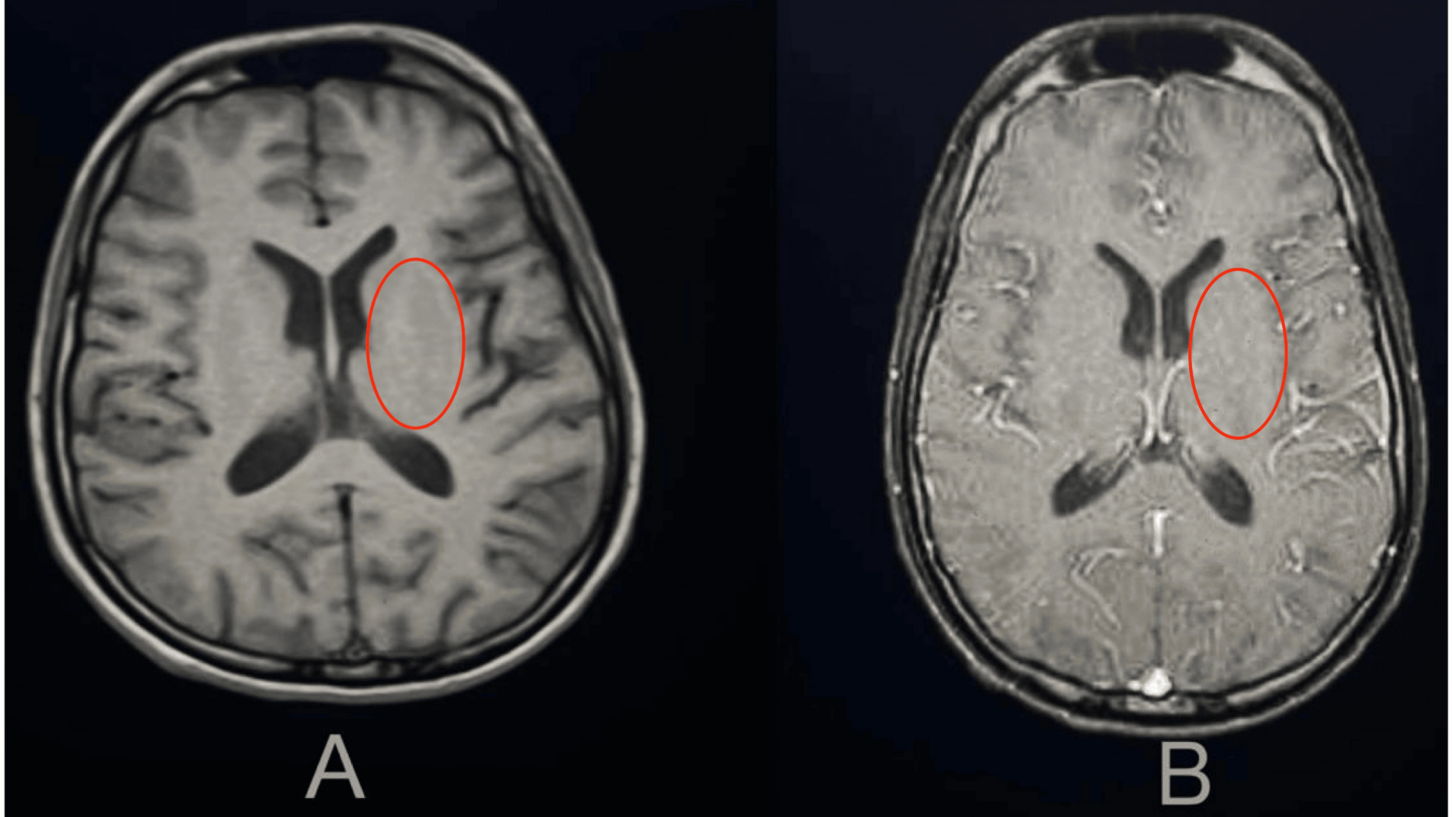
Brain MRI image cuts at the basal ganglia and caudate nucleus level A: Pre-contrast. B: Post-contrast. The red oval highlights the basal ganglia and caudate nucleus in both images. MRI: magnetic resonance imaging
